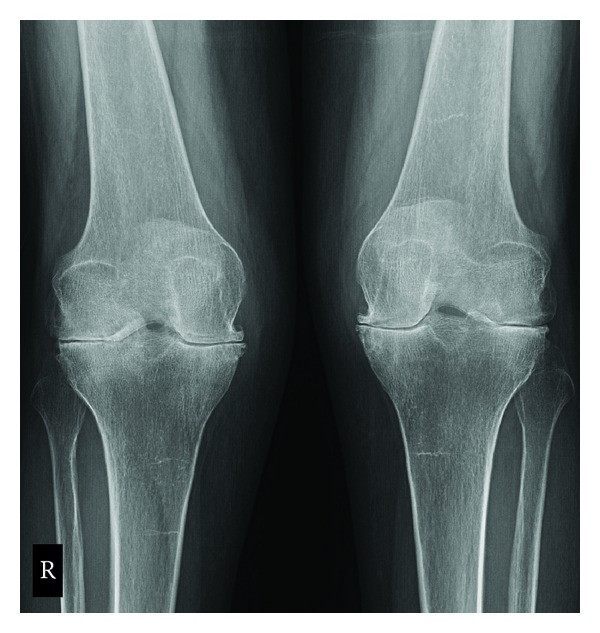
Posteroanterior radiographic view of bilateral knees demonstrating advanced arthritis. Note the valgus alignment to both legs. RA and OA radiographs differ in that RA radiographs will show periarticular erosions and osteopenia, whereas periarticular osteophytes, subchondral sclerosis, and joint space narrowing are more common in OA.
